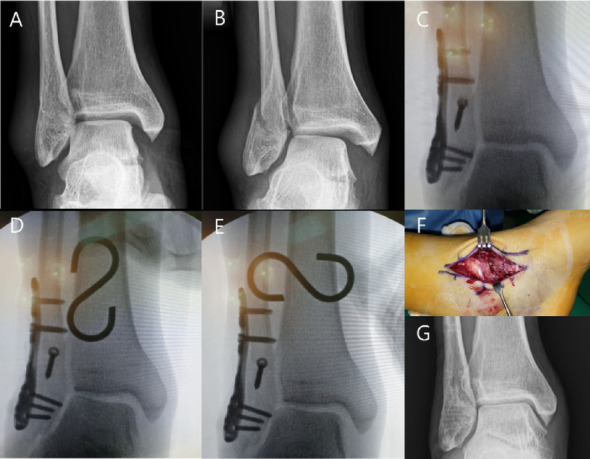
Representative radiographs and an intraoperative photograph of a 49-year-old man with a lateral malleolar fracture. A Preoperative anteroposterior ankle radiograph. B Preoperative gravity stress radiograph. C Intraoperative fluoroscopic image after lateral malleolar fixation. D Intraoperative valgus stress view. E Intraoperative external rotation stress view. These stress views demonstrated persistent medial instability after fixation; therefore, deltoid ligament repair was performed. F Intraoperative gross photograph showing rupture of the deep deltoid ligament. G Standing anteroposterior ankle radiograph obtained 13 months postoperatively
